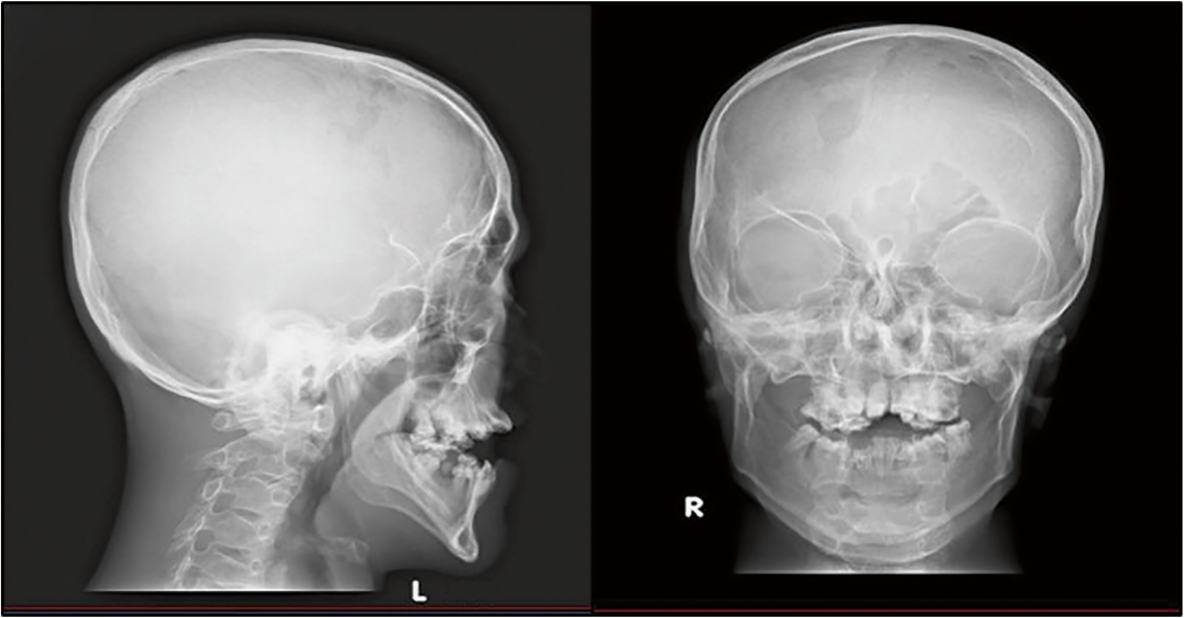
Craniofacial radiography of the patient. Radiographs showing facial dysmorphisms which include acrocephaly, asymmetrical flat facies, nasal deformity, and prominent jaw
